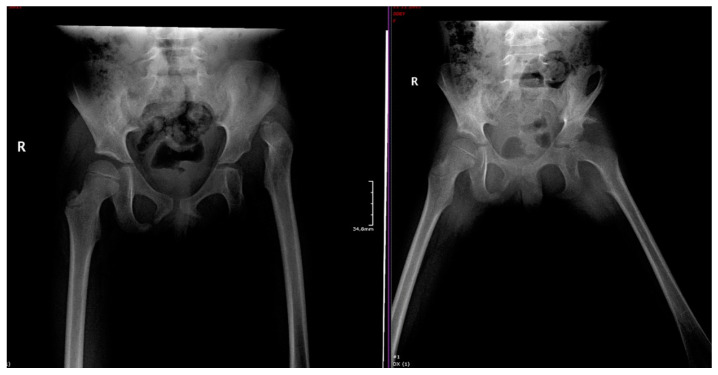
Proper acetabular orientation after a Salter osteotomy in a 5-year-old child. (Left) Pre-operative X-ray of the pelvis shows abnormal position of the femur head on the left side. (Right) Post-operative X-ray shows reorientation with good femoral head coverage and proper positioning of the bone graft. R: right side of patient; scale: 34.8 mm.
